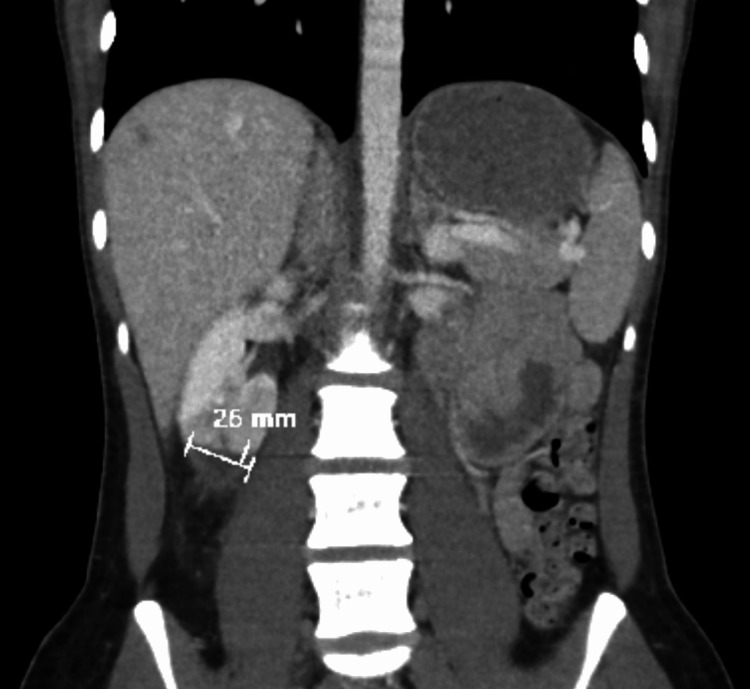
Coronal view of the contrast-enhanced abdominal computed tomography showing 26 mm right kidney infarction
